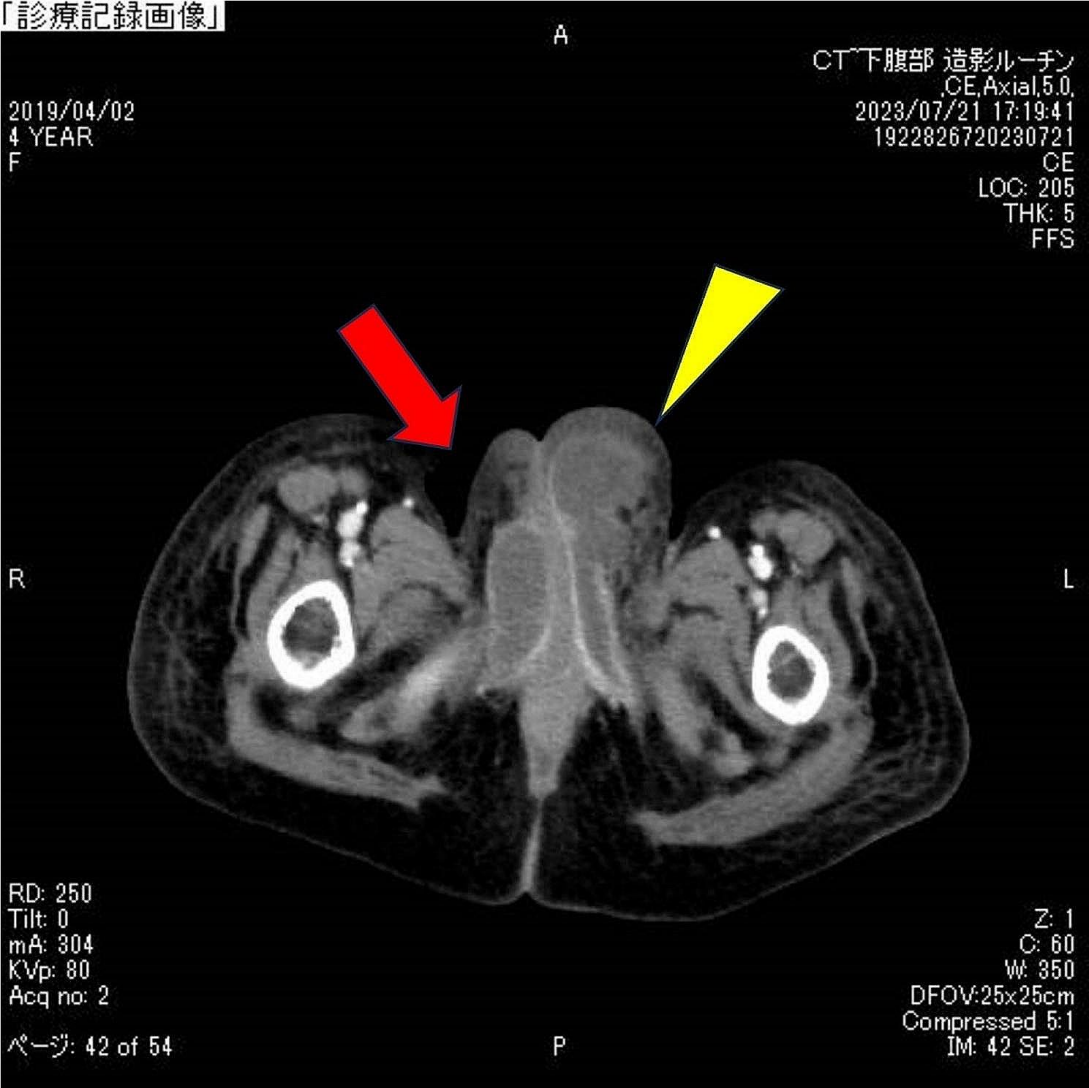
Contrast-enhanced computed tomography enhanced and swollen labia on both sides indicating bilateral bartholin’s gland abscesses (red arrow and yellow triangle)
